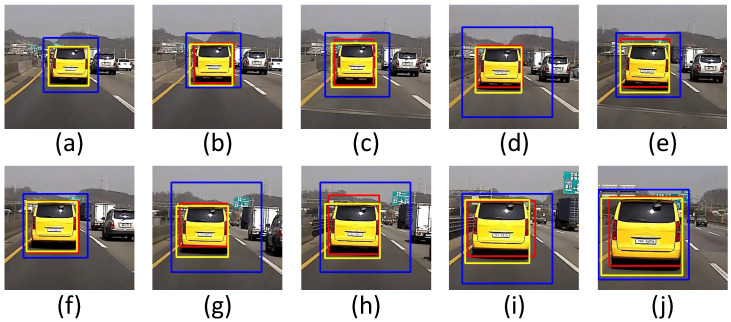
Vehicle detection results of the SUV: YOLOv3 (red), hybrid (yellow), and cascade (blue): (a) current frame-90. (b) current frame-80. (c) current frame-70. (d) current frame-60. (e) current frame-50. (f) current frame-40. (g) current frame-30. (h) current frame-20. (i) current frame-10. (j) current frame.
